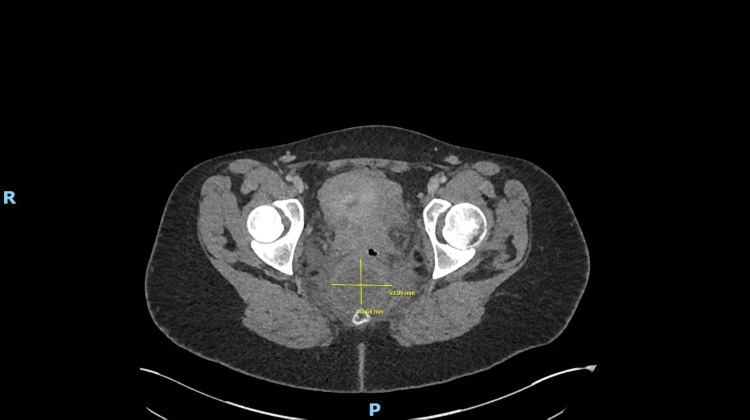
Axial CT pelvis (2020) showing lesion at initial diagnosis with adjacent structures
